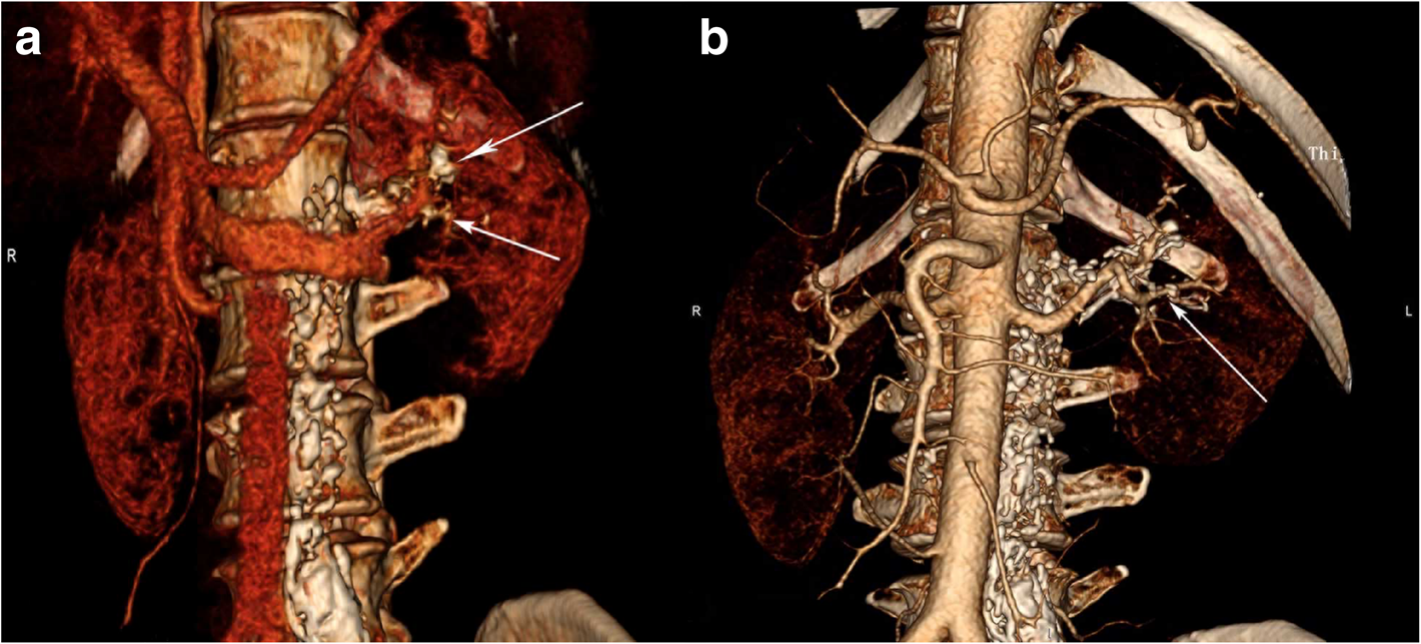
A 61-year-old woman with persistent idiopathic chyluria. a, b LPG CTA showing contrast agent adjacent to the left renal artery and its branches into the kidney
